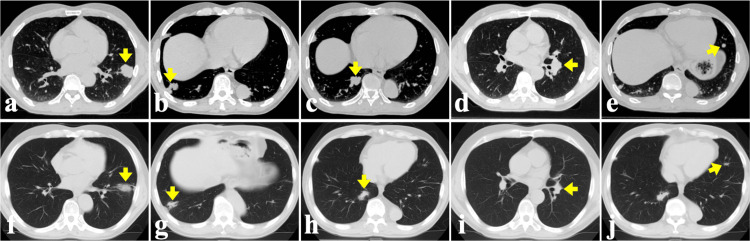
The lung metastases on plain CT before and after the CKR. (a)-(e) Five lung lesions before CKR. (f)-(j) Five lung lesions 6 months after CKR. CKR: CyberKnife radiotherapy.
